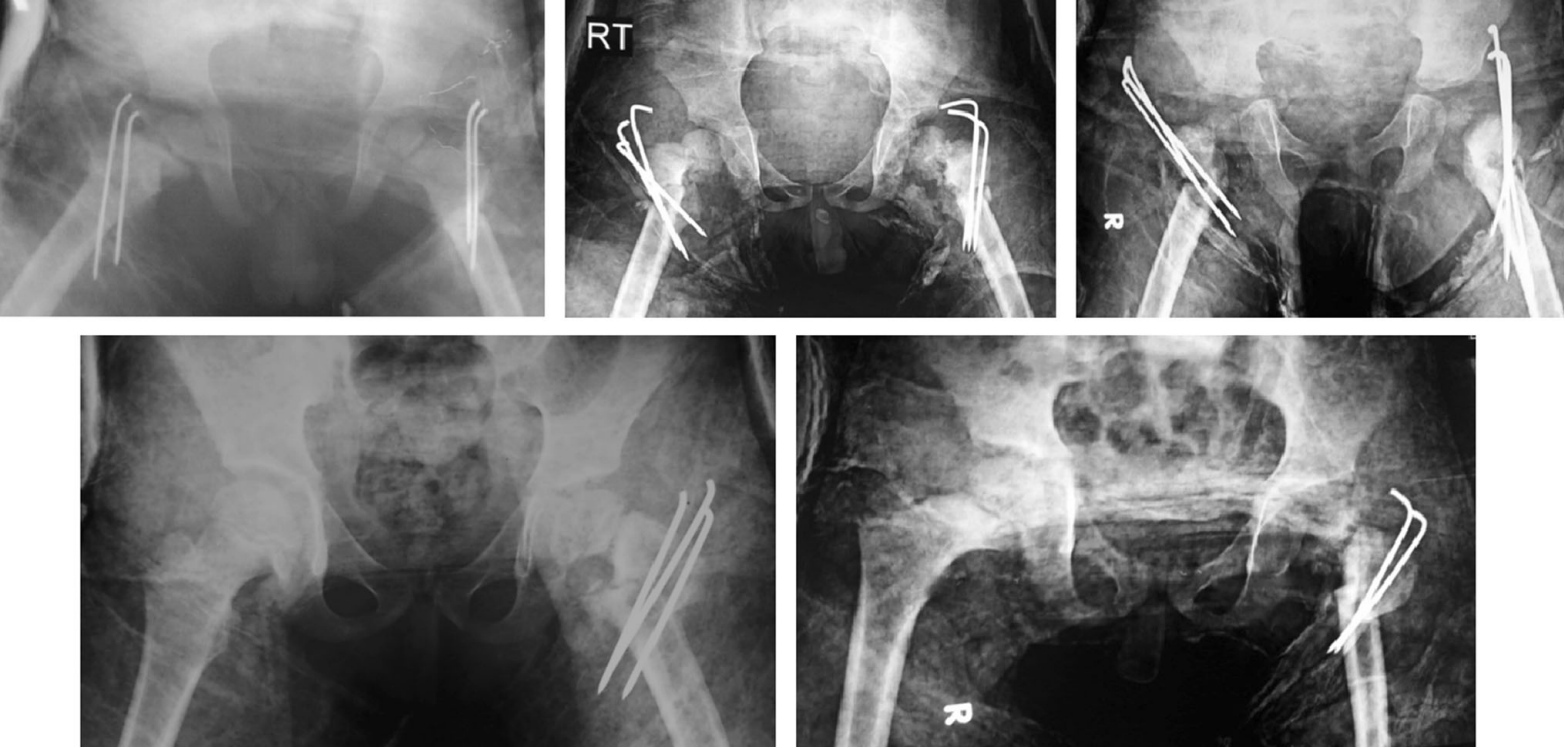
Immediate-postoperative X-rays of the hip spica casts, showing the correction achieved and the osteotomy fixation
